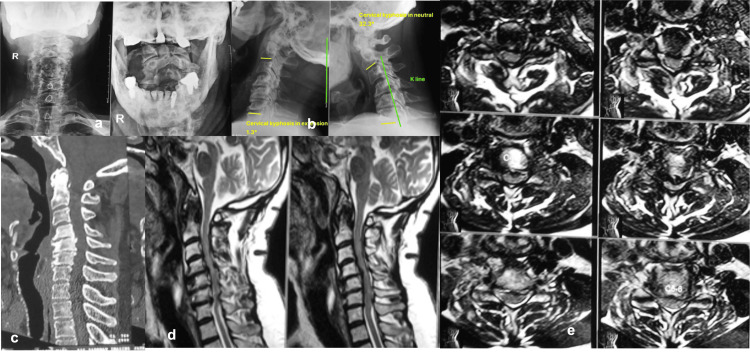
Preoperative pictures of a male patient with cervical myelopathy Preoperative pictures of a male patient with cervical myelopathy shows a) AP and Lateral X-ray, b) lateral flexion-extension X-rays, c) CT scan sagittal view showing disc-osteophyte complex with kyphosis, d) T2 sagittal view of MRI showing cervical stenosis and e) T2 axial view from C3-C7 levels showing narrowing of spinal canal diameter.
